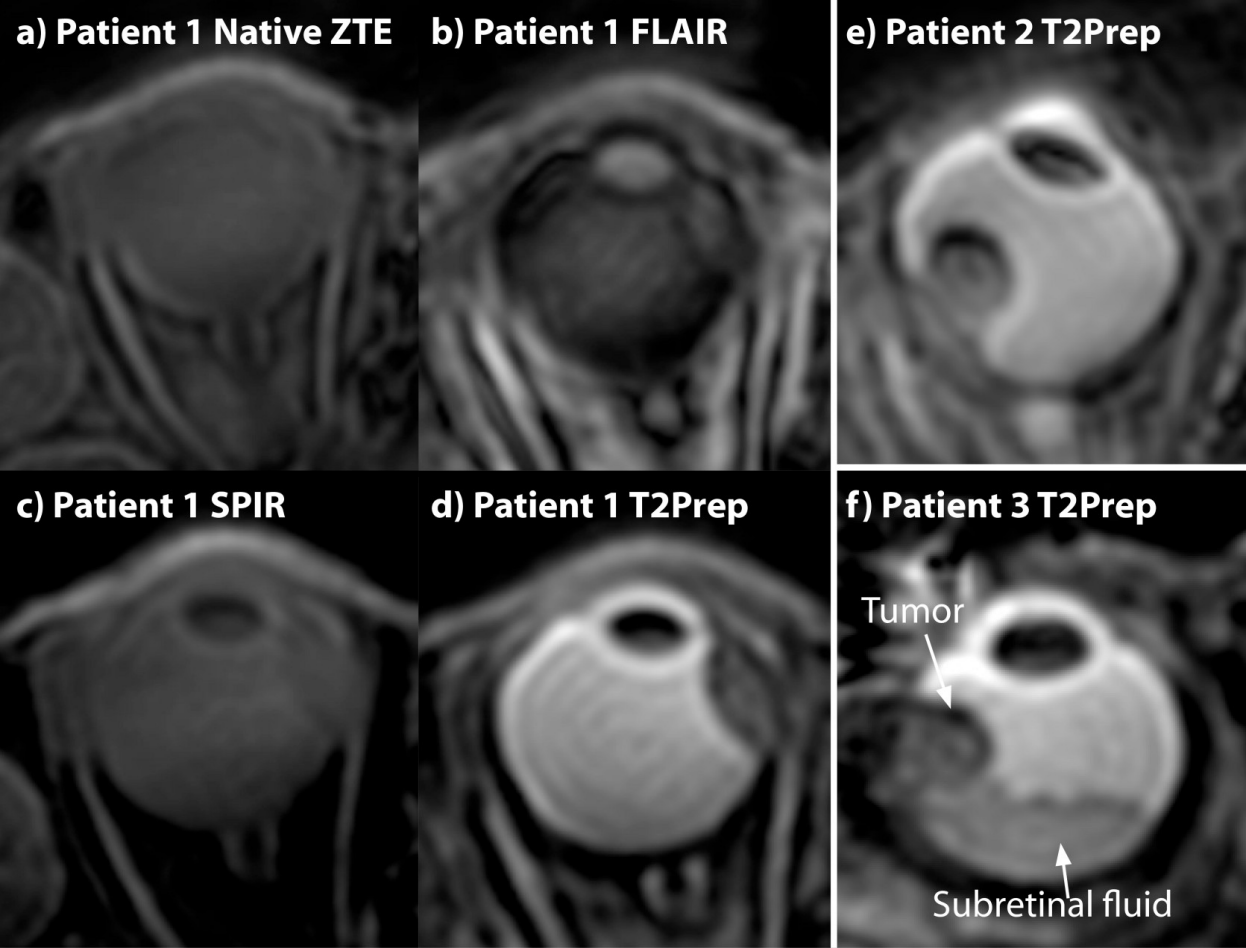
Magnetization-prepared ZTE imaging of the eye of three uveal melanoma patients. (a-d) ZTE images showing different magnetization preparation of one of the patients (isotropic (1mm^3^) resolution). In the native and fat suppressed ZTE the tumor cannot be discriminated from the surrounding tissues. The FLAIR ZTE does result in some degree of contrast between the vitreous body, but the tumor boundaries are not clearly identifiable as not all the signal from the vitreous body is suppressed. The T_2_-prepared ZTE, however, does provide a good contrast in which the tumor boundaries are clearly visible. (e,f) T_2_-prepared ZTE scans of two other patients, showing clearly the tumor in the eye. The third patient (f) furthermore suffers from a retinal detachment, a common complication of an ocular tumor, which results in fluid accumulation behind the retina. This subretinal fluid can be identified as a region which has a signal intensity between the tumor and the vitreous body.
